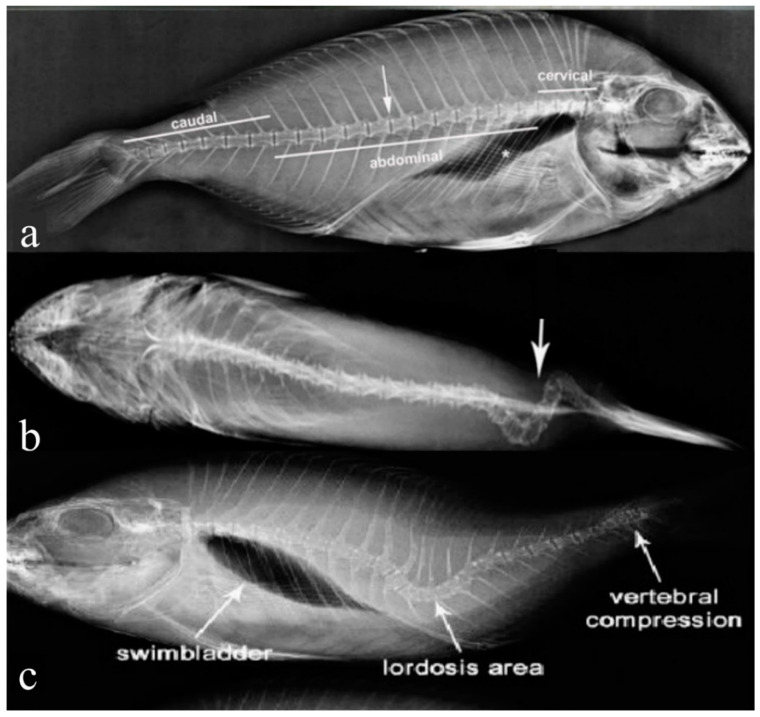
X-ray of gilthead seabream with different forms of spinal deformities. (a) Normal anatomy of cervical, abdominal, and caudal regions of the vertebral column. Inter-vertebrae space is highlighted with an arrow, and swim bladder is indicated with a star; (b) apical X-ray of seabream caudal region with scoliosis (highlighted with arrows); (c) X-ray of seabream with coexisting two main deformities of the vertebral column lordosis and vertebral compression. (a,b) Are adapted from Boursiaki et al., 2019 [48], while (c) is adapted from Berellis et al., 2015 [49].
